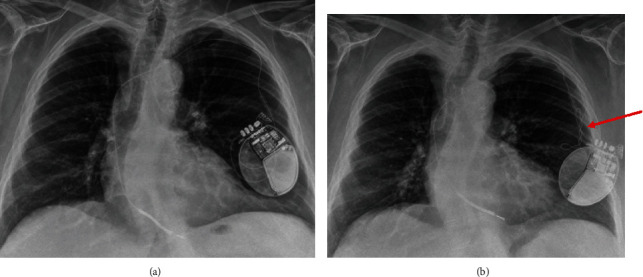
PA chest X-ray one month postimplantation (a) and 3 years postimplantation showing twisting of the lead (b) (red arrow).
